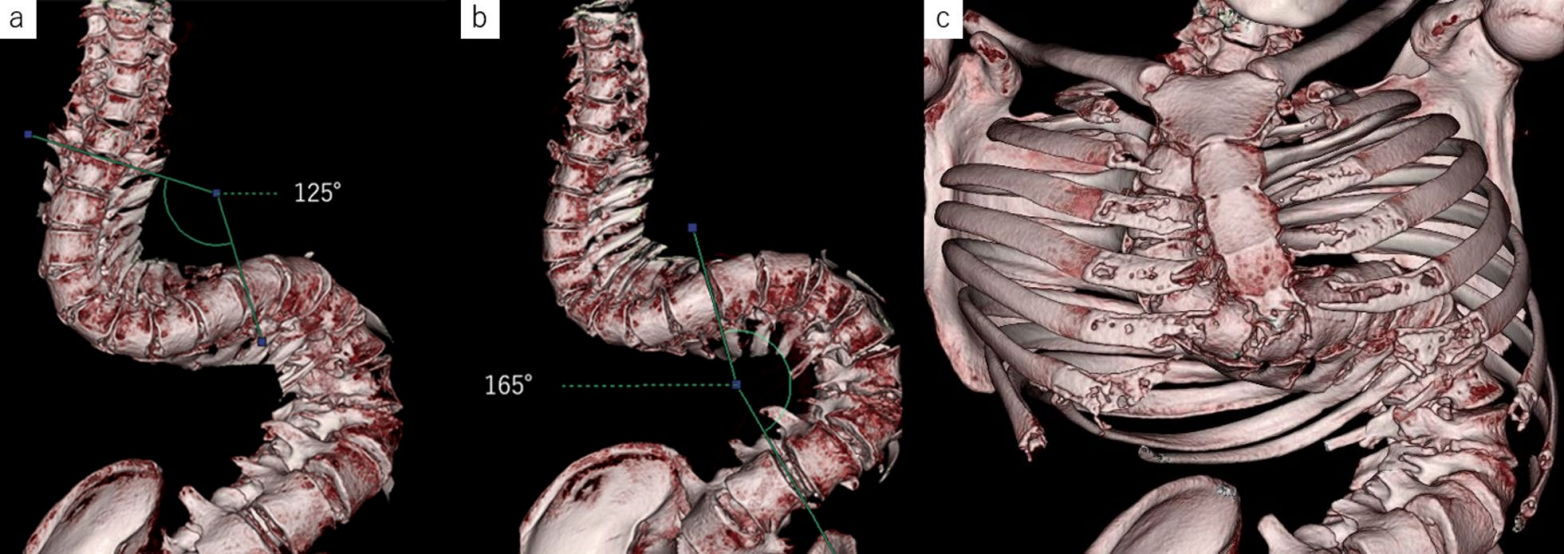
The patient had severe scoliosis. The spinal curve was convex to the right at the level of the middle thoracic esophagus, and convex to the left at the level of the lower esophagus, with Cobb angles of 125° and 165°, respectively (a, b). 3D-CT image of the spine and ribs shows the narrowing of the rib cage (c)
